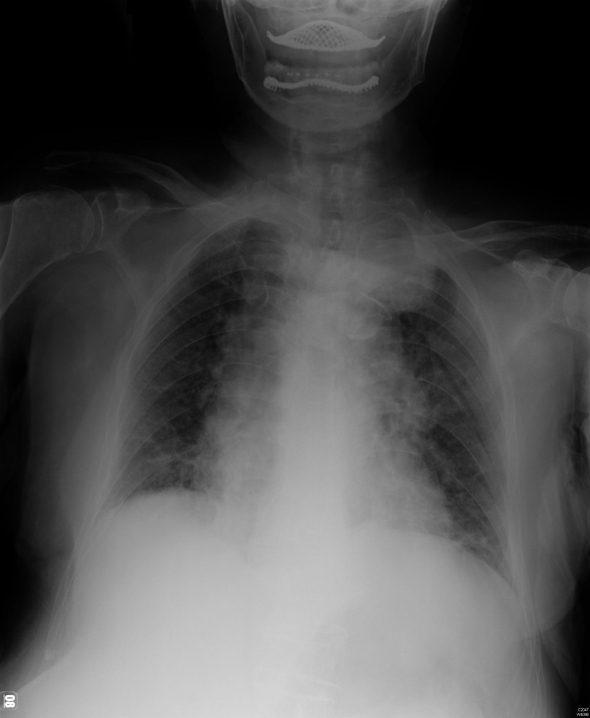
Chest radiography shows a soft tissue lesion at left apex.
